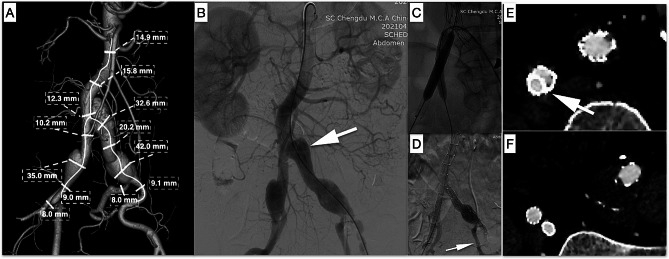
Case 1: A Preoperative CT imaging and aneurysm anatomical parameter measurements. The diameter of the right proximal CIA measured 10.2 mm. B Intraoperative angiogram. In the proximal left CIA, a dissection was observed (white arrow). C Two noncompliant 10 mm balloons were simultaneously inflated inside the parallel covered stents. D The final angiogram demonstrated successful deployment of Aegis™ and two parallel covered stents in the right iliac artery. The left IIA was embolized by coils (white arrow). E The cross-sectional image of the two-year follow-up CT scan demonstrated the “eye of the tiger” configuration (white arrow), with no evidence of gutter leaks. F On the same CT scan, the EIA and IIA stents were patent in the right iliac artery
